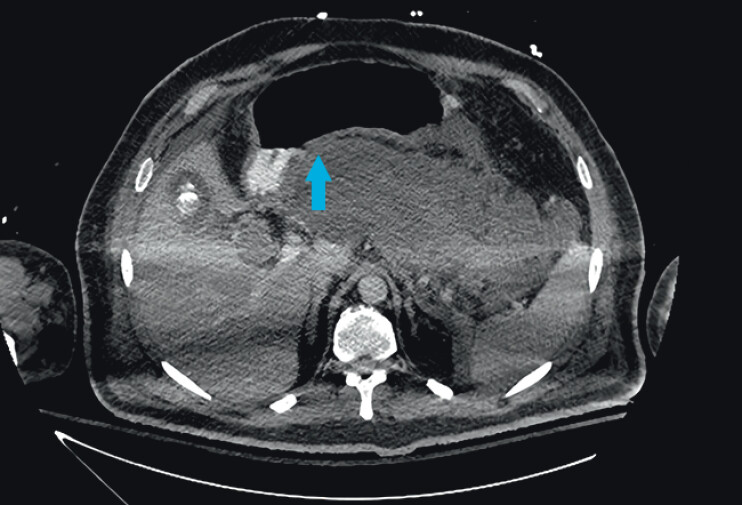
Computed tomography image showing the site of optimal contact (blue arrow) between the pancreatic collection and the gastric wall.
